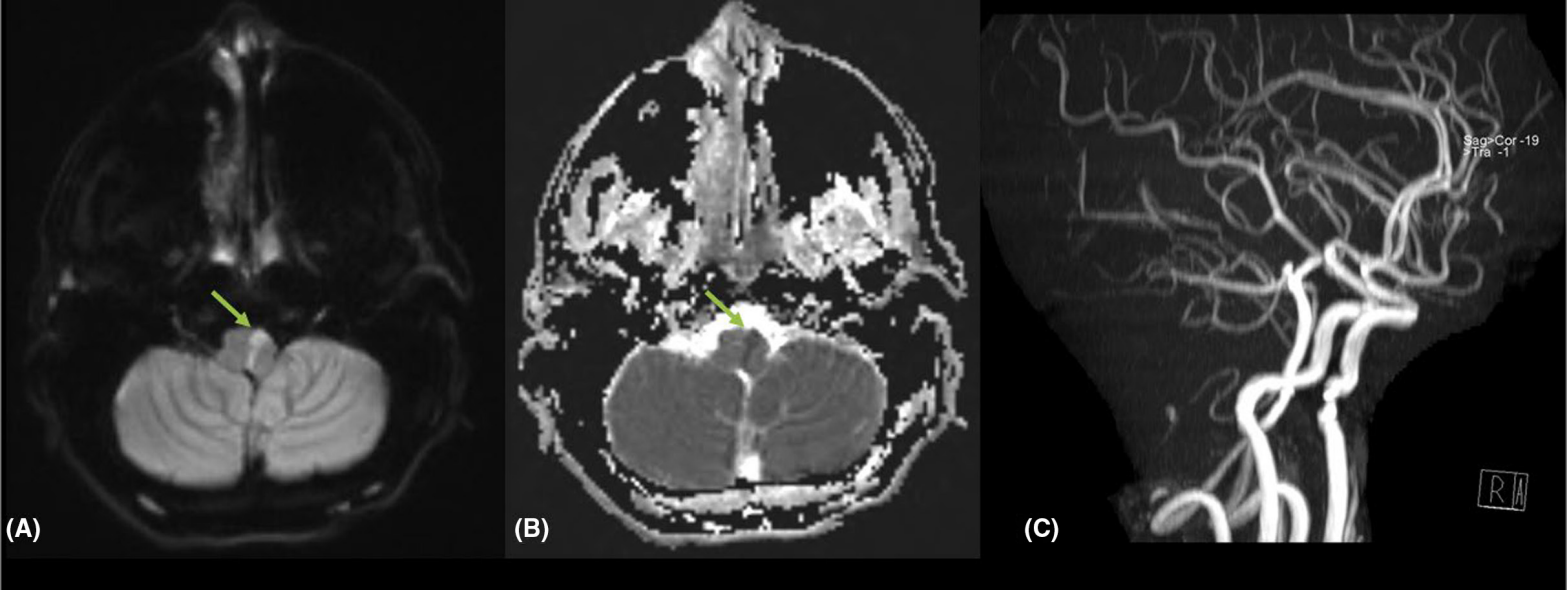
MRI head axial cuts: (A) DWI and (B) ADC, showed a linear area of diffusion restriction along the left paramedian aspect of the upper medulla oblongata with high signal intensity in diffusion‐weighted images (DWI) and low value in ADC map (green arrows) denoting recent ischemic infarction. There was no evidence of hemorrhagic components within. (C) Intracranial MRA was unremarkable with no major vascular occlusion, stenosis, aneurysm, or AVM.
